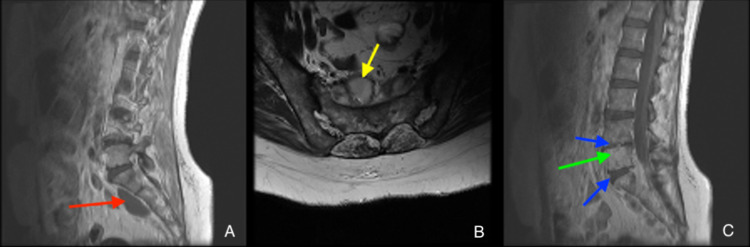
MRI upon admission. Contrast-enhanced sagittal T1 (A), axial T2 (B), and sagittal STIR (C) showing osteodiscitis centered around L5-S1 (green arrow), loss of disc height at L4-L5 and L5-S1 (blue arrows), rim-enhancing abscess anterior to the sacrum (red arrow), and rim-enhancing lesion in the right piriformis (yellow arrow). MRI: magnetic resonance imaging; STIR: short tau inversion recovery
